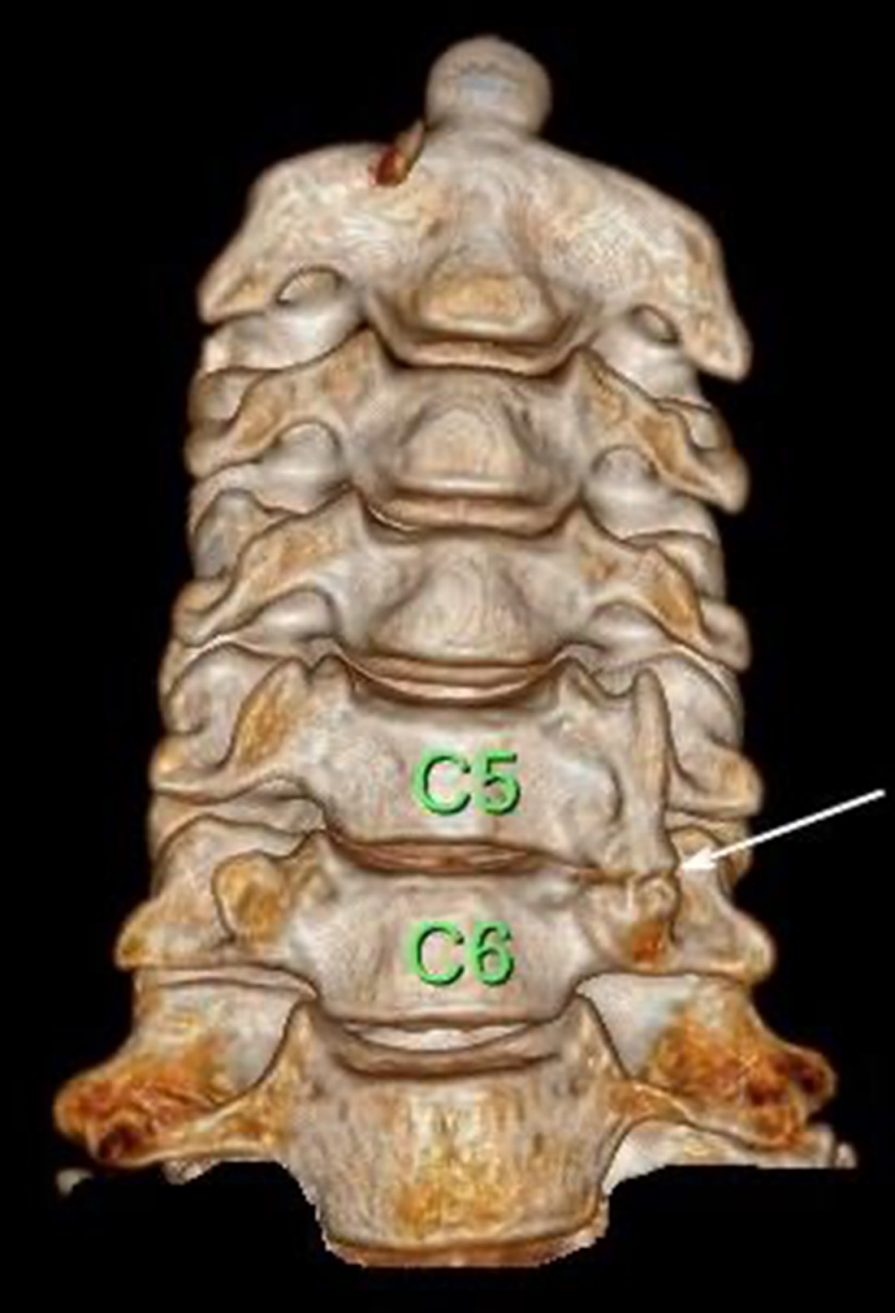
Coronal volume-rendered CT images clearly show the accessory articulation between the C5/C6 transverse processes without any associated fractures.
